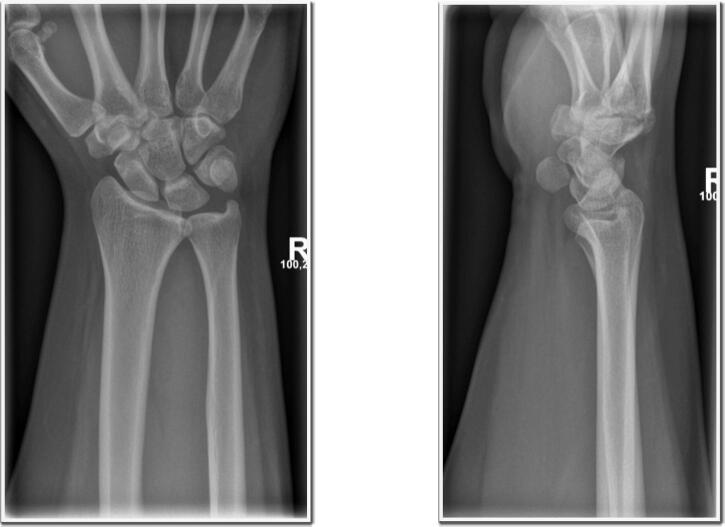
Radiographic evidence of anterior pisiform dislocation in the right hand (Universitätsmedizin Halle, Saale).
